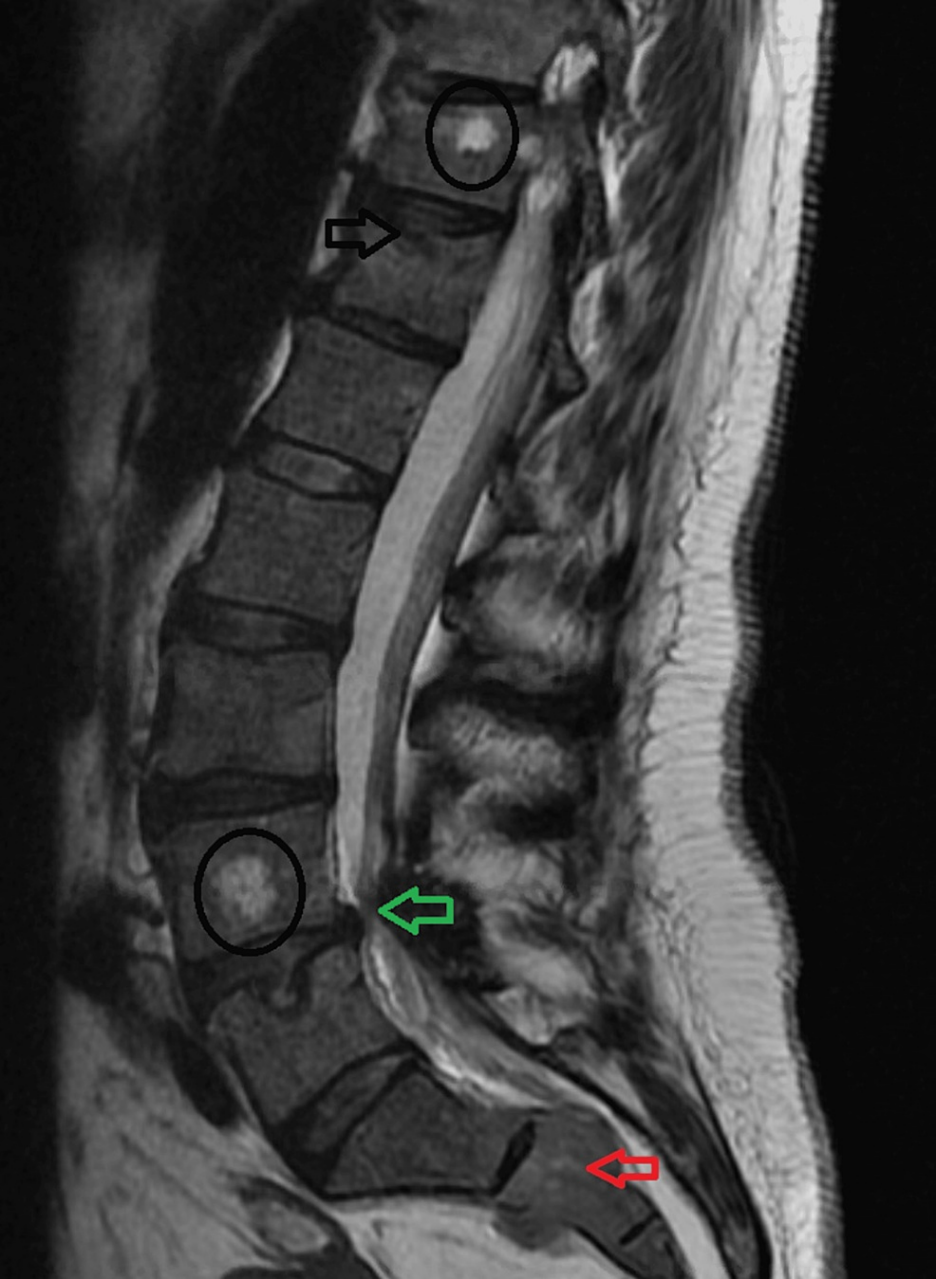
T2-weighted sagittal magnetic resonance imaging of the lumbar spine. *Black circles*: Multiple metastatic lesions identified in the thoracic and lumbar vertebral column. *Black arrow:* Wedge compression fracture of T12 vertebral body. *Green arrow:* Central canal stenosis at the L4-L5 intervertebral space. *Red arrow:* Large lytic lesion identified at the S2 level.
